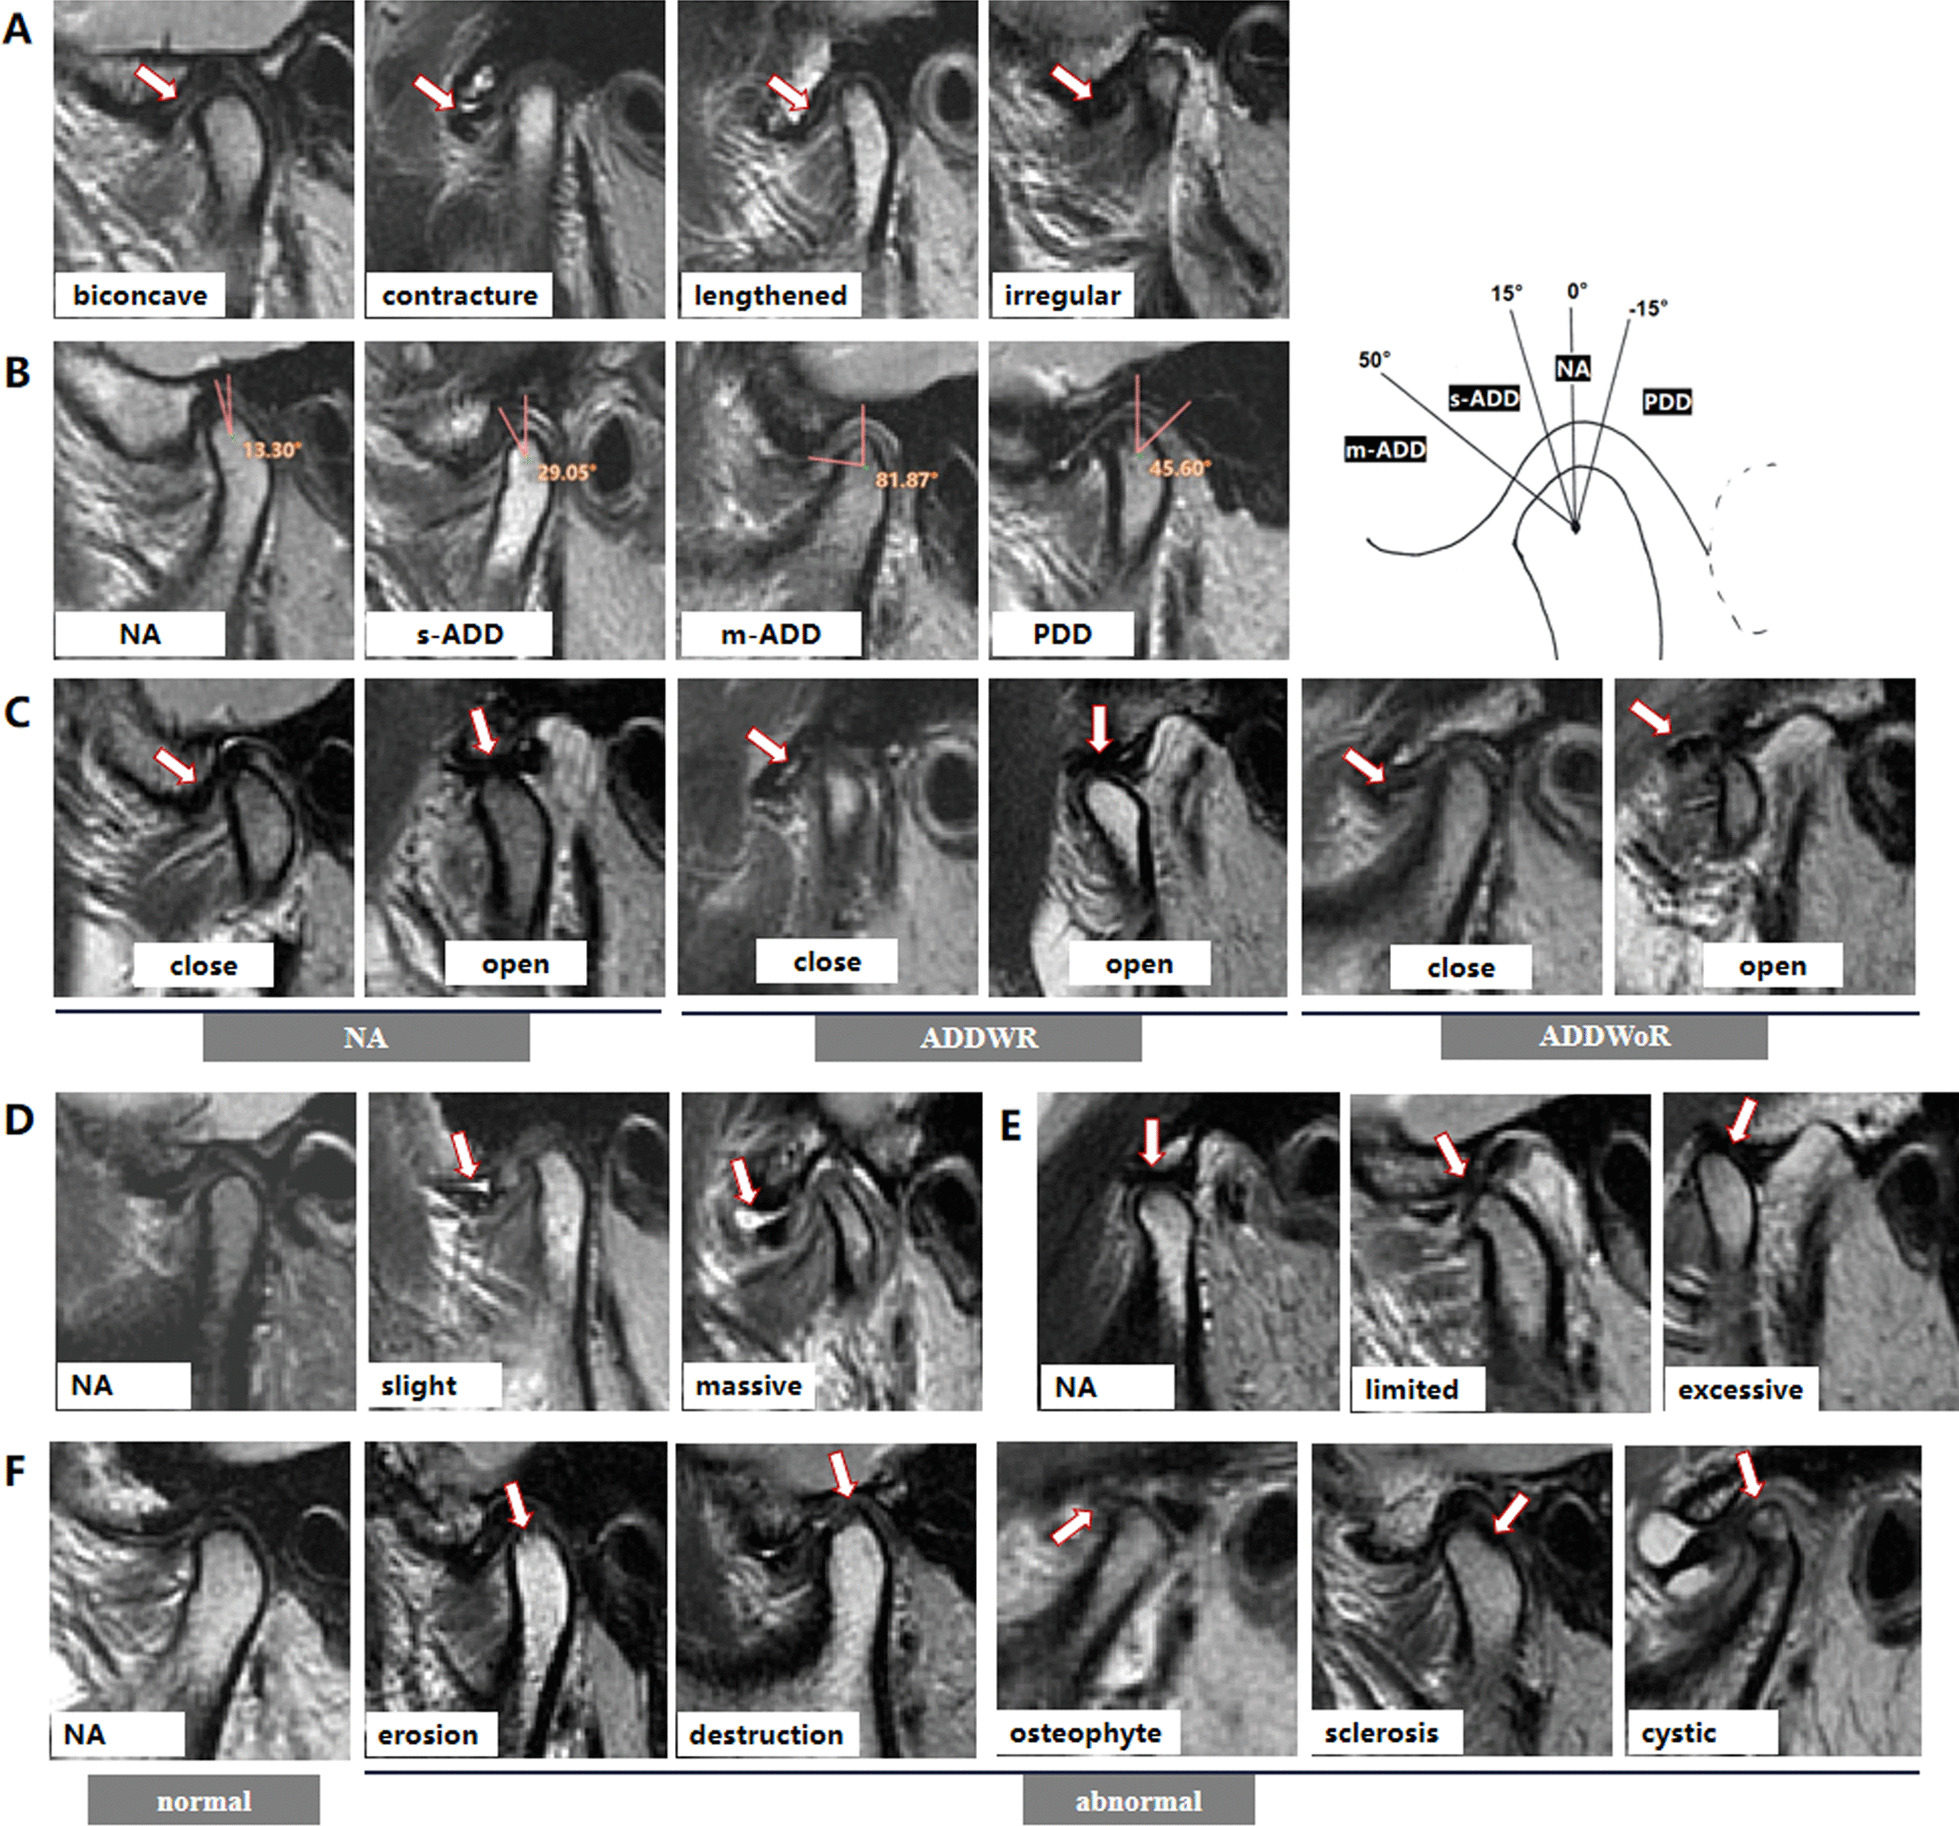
MRI imaging. A The shape of disc (the arrow indicates the articular disk); B the position of disc (the Angle between the posterior band and the condylar head in the closed-mouth position); C classification of anterior articular disc displacement (depending on the position of the articular disc in the opening position); D joint effusion (the arrow indicates the effusion); E joint motion (the position of the condyle with respect to the articular tubercle in the opening position); F condylar bone morphology (the arrow indicates the bone abnormality). Where: NA = normal; s-ADD = slight to mild anterior disc displacement; m-ADD = moderate to severe anterior disc displacement; PDD = posterior disc displacement; ADDWR = anterior disc displacement with reduction; ADDWoR = anterior disc displacement without reduction
